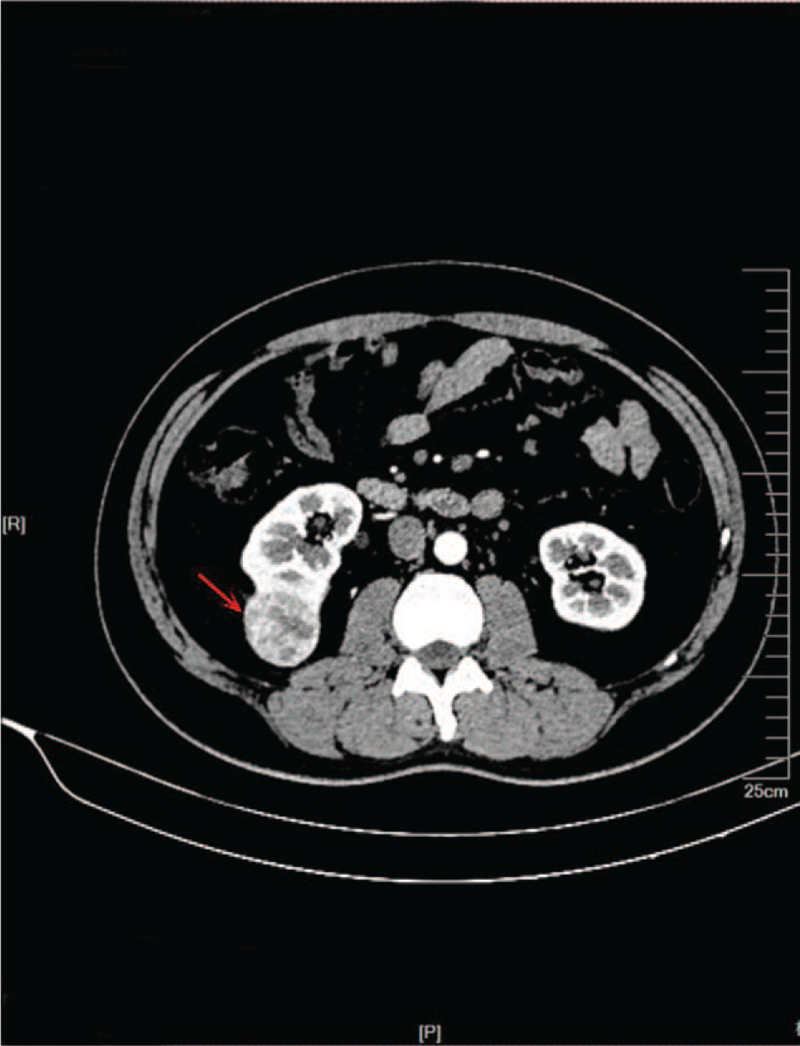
CT abdomen: A quasi-circular slightly high-density mass in the lower part of the right kidney, with calcification in the lower part of the right kidney, protruding the outline of the kidney and showing obvious inhomogeneous enhancement in the arterial phase.
